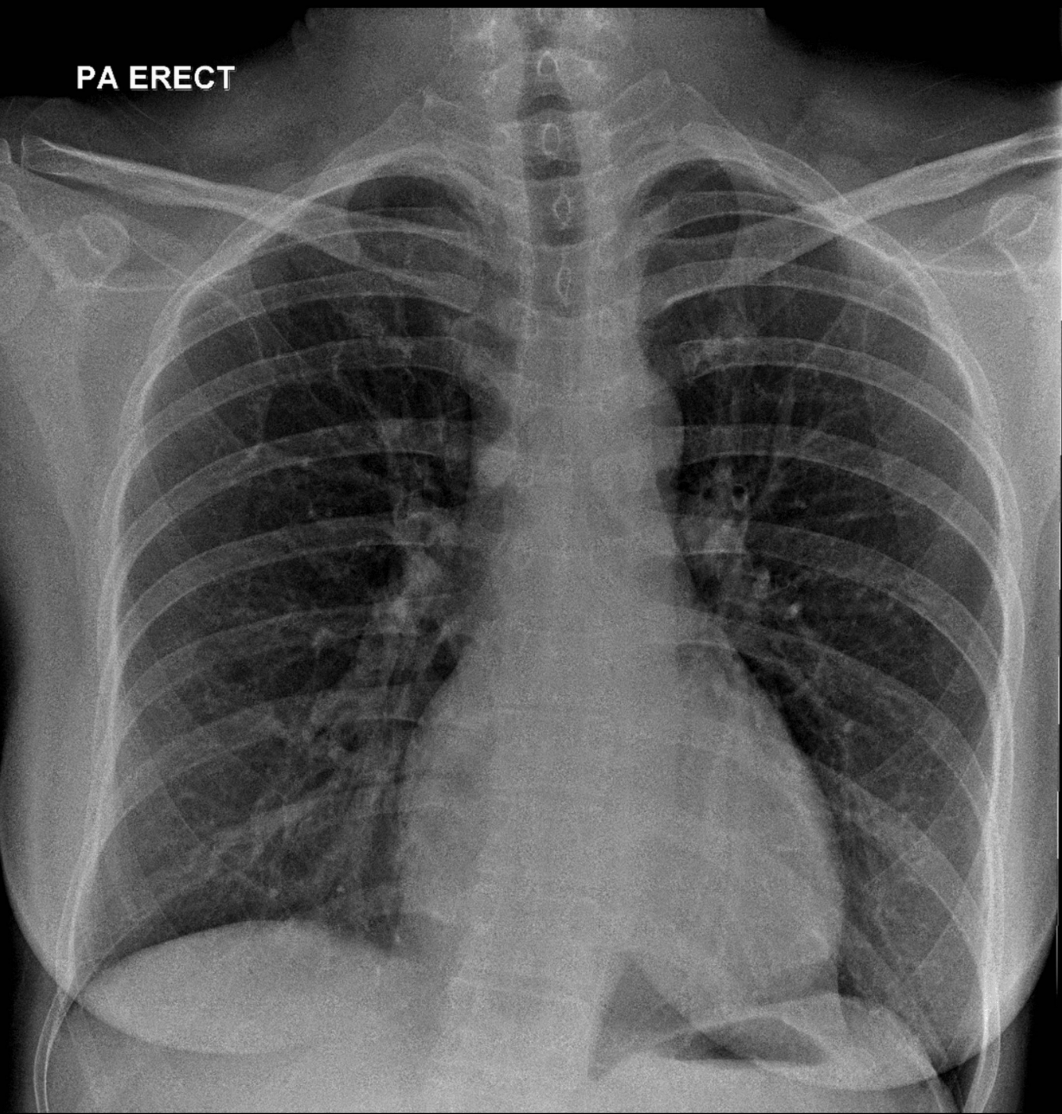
Supplementary chest radiograph did not show radioopacity at the ROI. ROI: Region of interest
